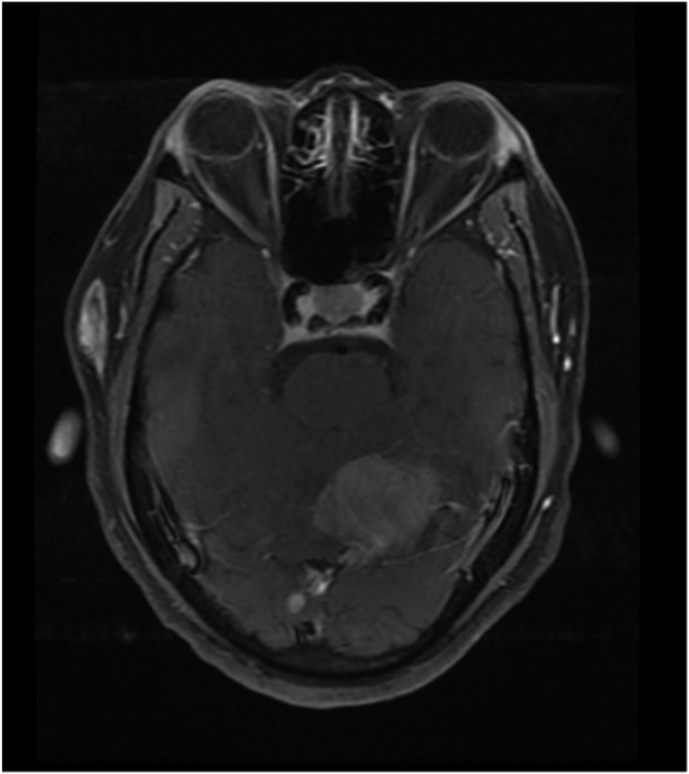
Brain (head) magnetic resonance imaging (MRI). Multiple intra-axial expansive lesions diffused through the supra and infratentorial compartments, with emphasis on lesions in the nucleus capsular region, mesencephalic transition, and in the periphery of the right cerebellar hemisphere, determining an expansive effect with a deviation of the contralateral cerebellar vermis.
